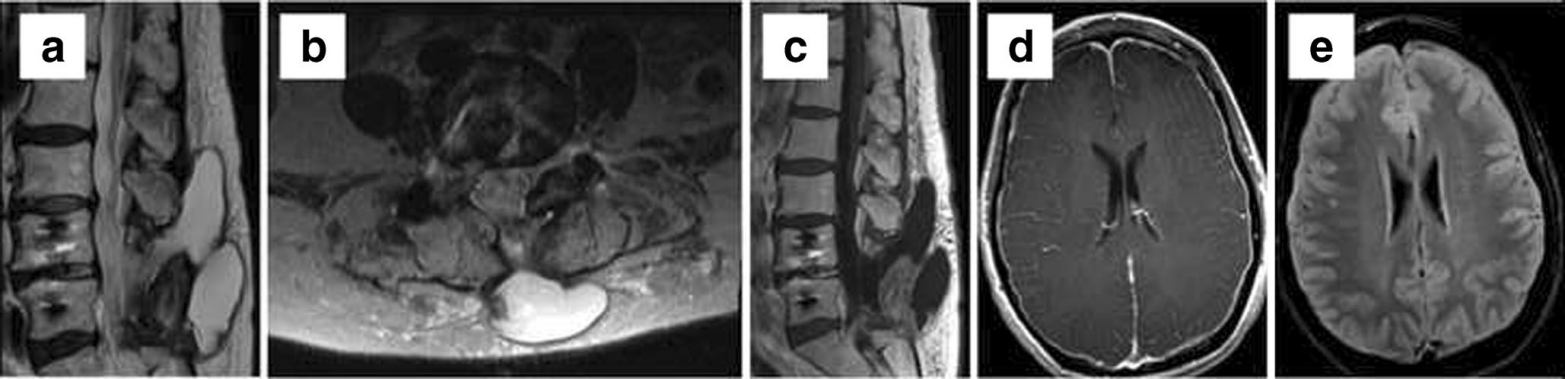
Sagittal and axial T2 WIs (a and b) show a large epidural and posterior paraspinal fluid collection in a patient with L4–5 decompression and fusion. Postcontrast image (c) shows no significant enhancement around the collection. The patient had orthostatic headaches and MRI brain confirmed dural thickening and effusion on FLAIR (d) with diffuse pachymeningeal enhancement (e) consistent with intracranial hypotension due to CSF leak and psedomeningocele formation
